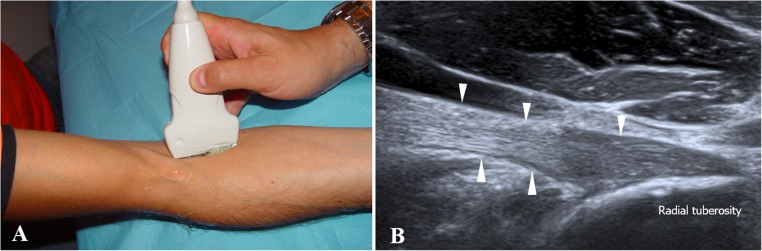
Long-axis oblique ulnar approach. a The patient is sitting on a stool in front of the examination table with the shoulder in external rotation, elbow in slight flexion and forearm in full supination. The transducer is placed on the midline of the elbow, at about 20° of ulnar rotation in relation to the upper limb long axis and tilted about 30° medially. b Ultrasound image obtained using the approach described in a. The fibrillary echotexture of the DBBT (*white arrowheads*) at the attachment site to the radial tuberosity is observed
